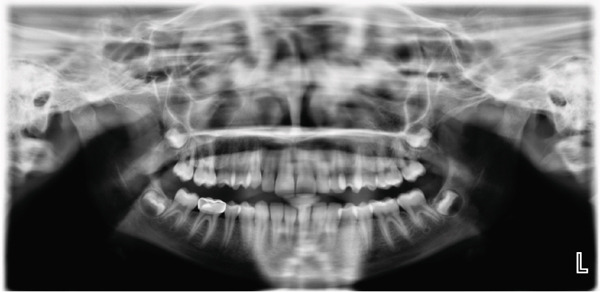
Posttreatment radiographic documentation: 24‐month panoramic radiograph.
